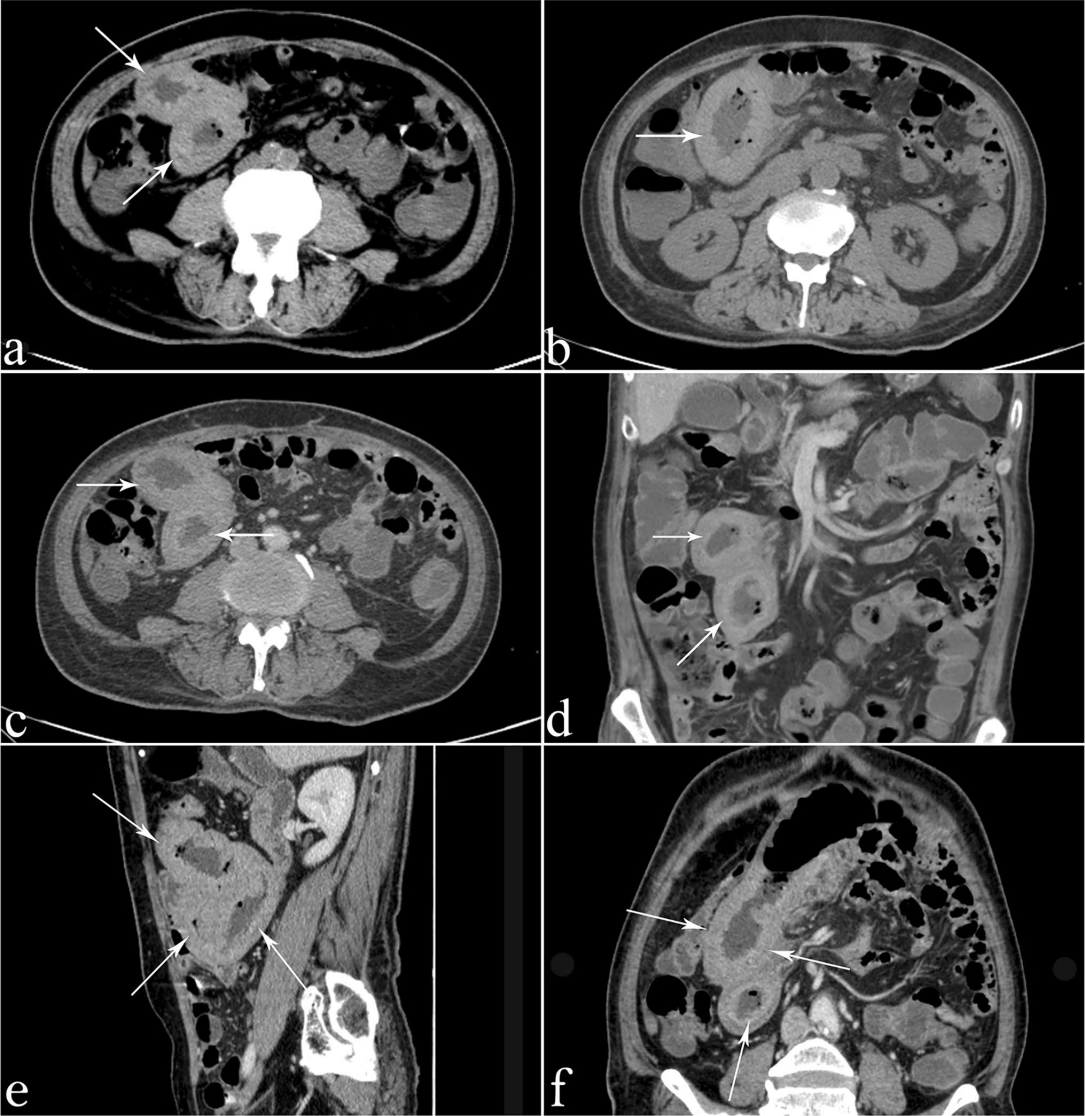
A 46-year-old male patient with adhesive SBO with SBN had undergone appendicitis surgery more than 10 years ago, with symptoms of abdominal pain, nausea, vomiting, and fever. a Two thickened and high-density small-bowel walls with the target sign (arrows) can be seen on CT plain scanning, and the CT value of the small-bowel wall on plain scanning is approximately 53 HU. b The arrow shows the thickened small-bowel wall with a high-density target sign at different levels. c After enhanced scanning, the thickened small-bowel wall with a high-density target sign shows a decrease in enhancement. After enhancement, the CT value of the small-bowel wall is 57 HU, with almost no enhancement. d and e The coronal and sagittal views show a thickened small-bowel wall with the high-density target sign. f The target sign can be seen in the image with 3D MPR (arrow)
